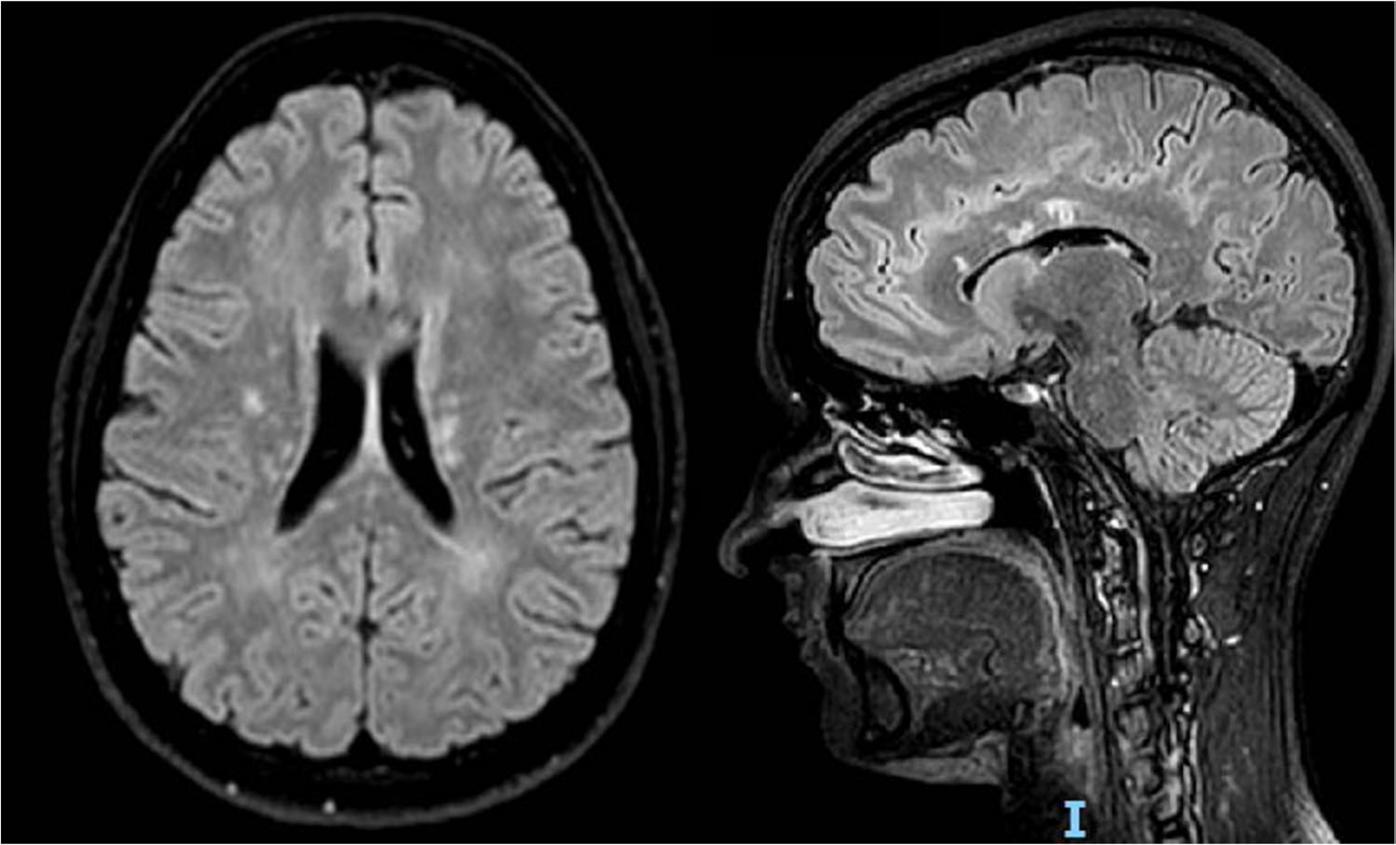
Brain MRI showing FLAIR hyperintense white matter lesions with "snowball"-like lesions in the corpus callosum
